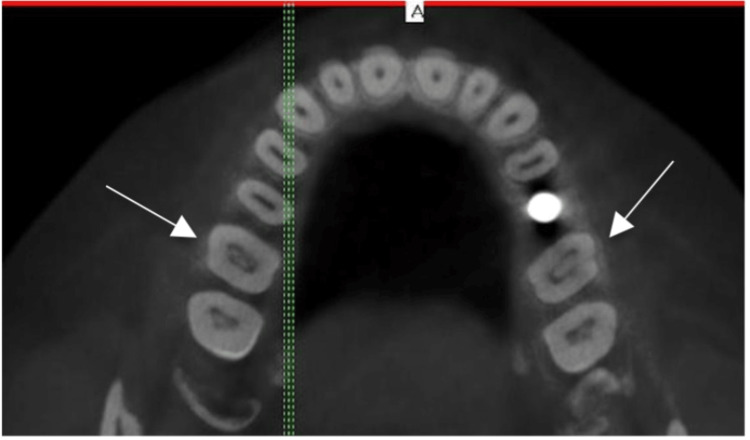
CBCT axial section showing pulp stones in the upper right and left first molars CBCT: cone-beam computed tomography
